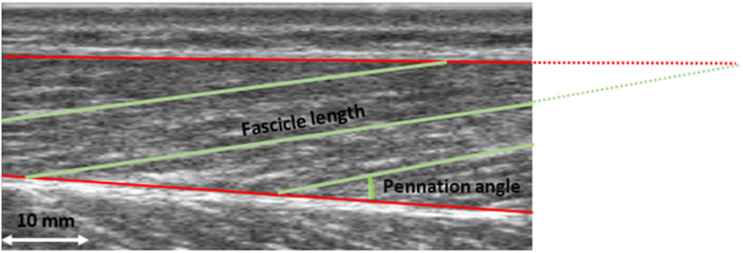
Ultrasound image of the m. vastus lateralis. Fascicle length and pennation angle were determined for three fascicles. Pennation angle was calculated between the muscle fascicle and the deep aponeurosis. Fascicle length was defined as the distance between the intersection of the upper aponeurosis with the muscle fascicle and the intersection of the lower aponeurosis and muscle fascicle. The mean of the three measured values was used for further analysis.
